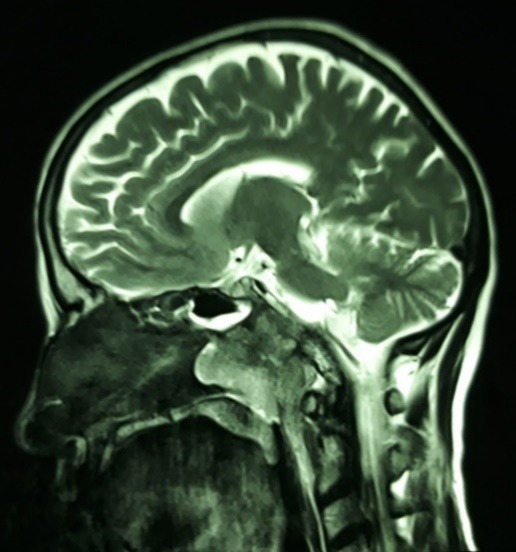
Brain and cervical MRI, T2 weighted, sagittal cut: heterogeneously enhancing mass in the right aspect of the nasopharynx
